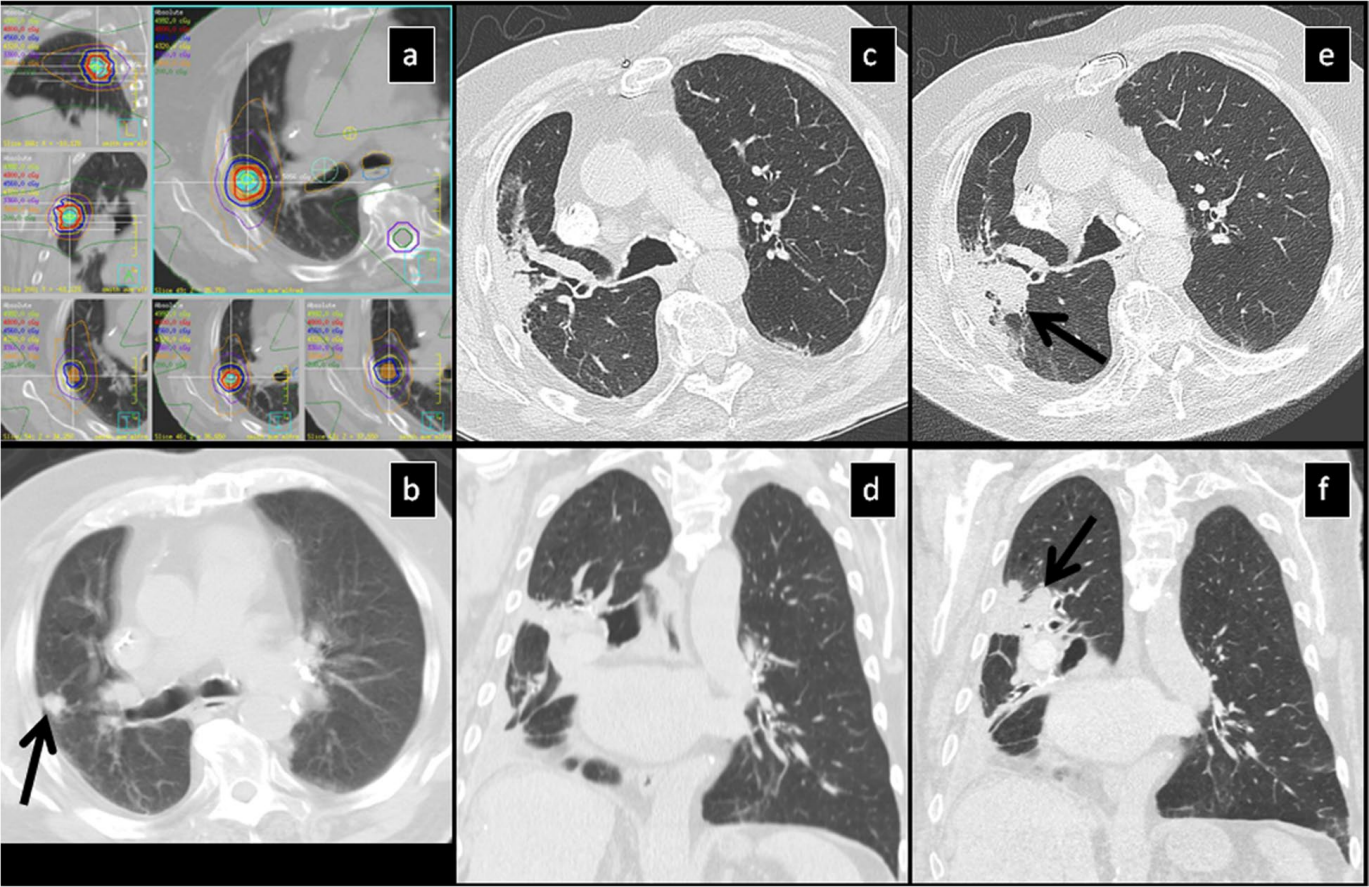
SBRT-treated lung cancer demonstrates local recurrence at 5 years: a) Isodose multiplanar images for planning. b) pre-SBRT, CT shows a solid nodule (black arrow). Axial (c) and coronal plane (d) chest CT 3 years post-SBRT show “modified conventional pattern” that has stabilized. 5 years post-SBRT axial (e) and coronal (f) images show increased soft tissue density bulging on one side of the lesion (posteriorly on axial plane and superiorly on coronal plane, black arrows) in keeping with local recurrence
